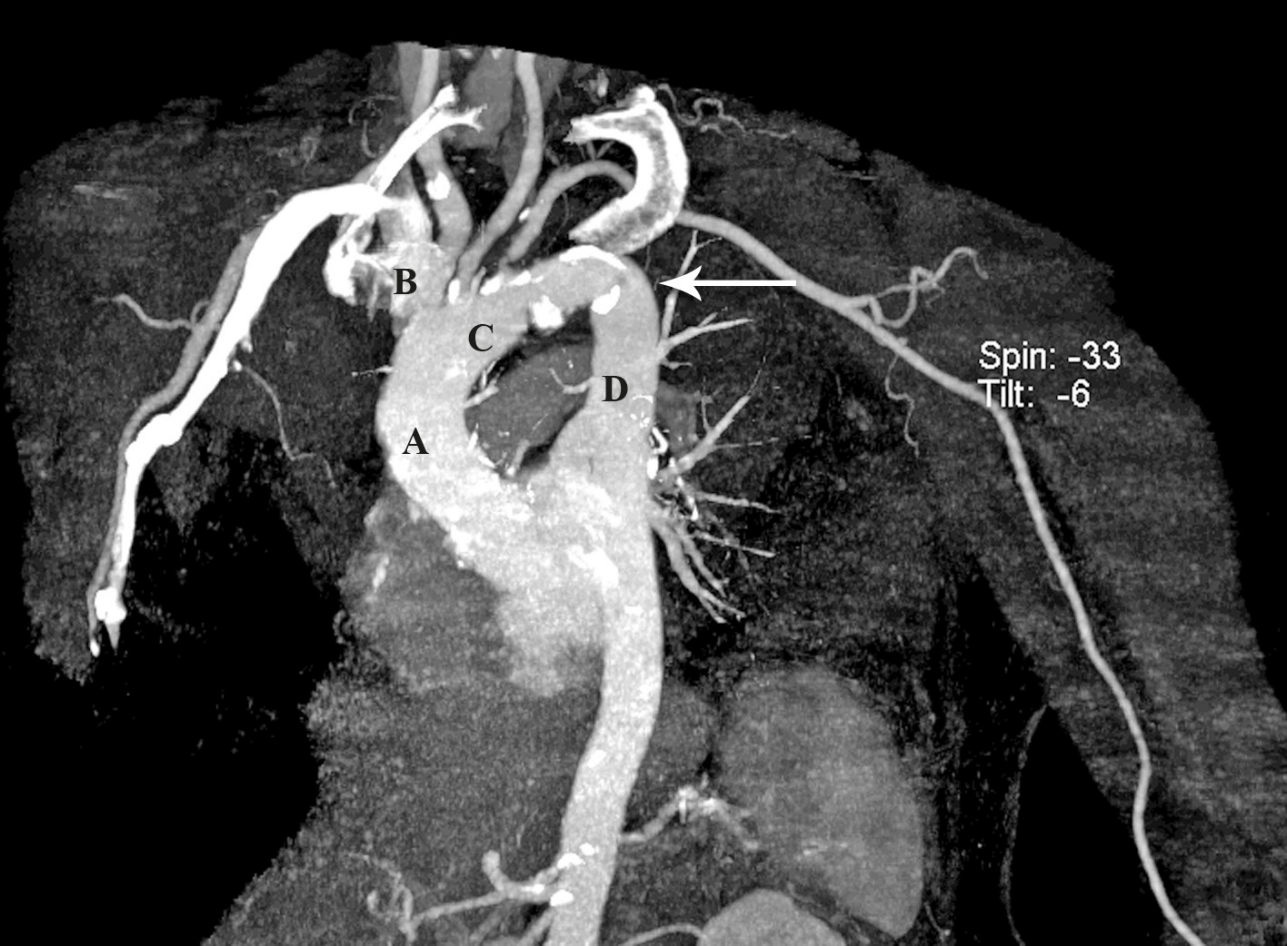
Computed tomography angiography of the aortic arch in the anteroposterior projection reveals calcified components of the aortic mass with density, similar to the adjacent bony structures. Letters A, B, C, and D stand for the ascending aorta, innominate artery, aortic arch, and descending thoracic aorta, respectively. Horizontal arrow shows calcified components of the aortic arch atherosclerotic plaque.
